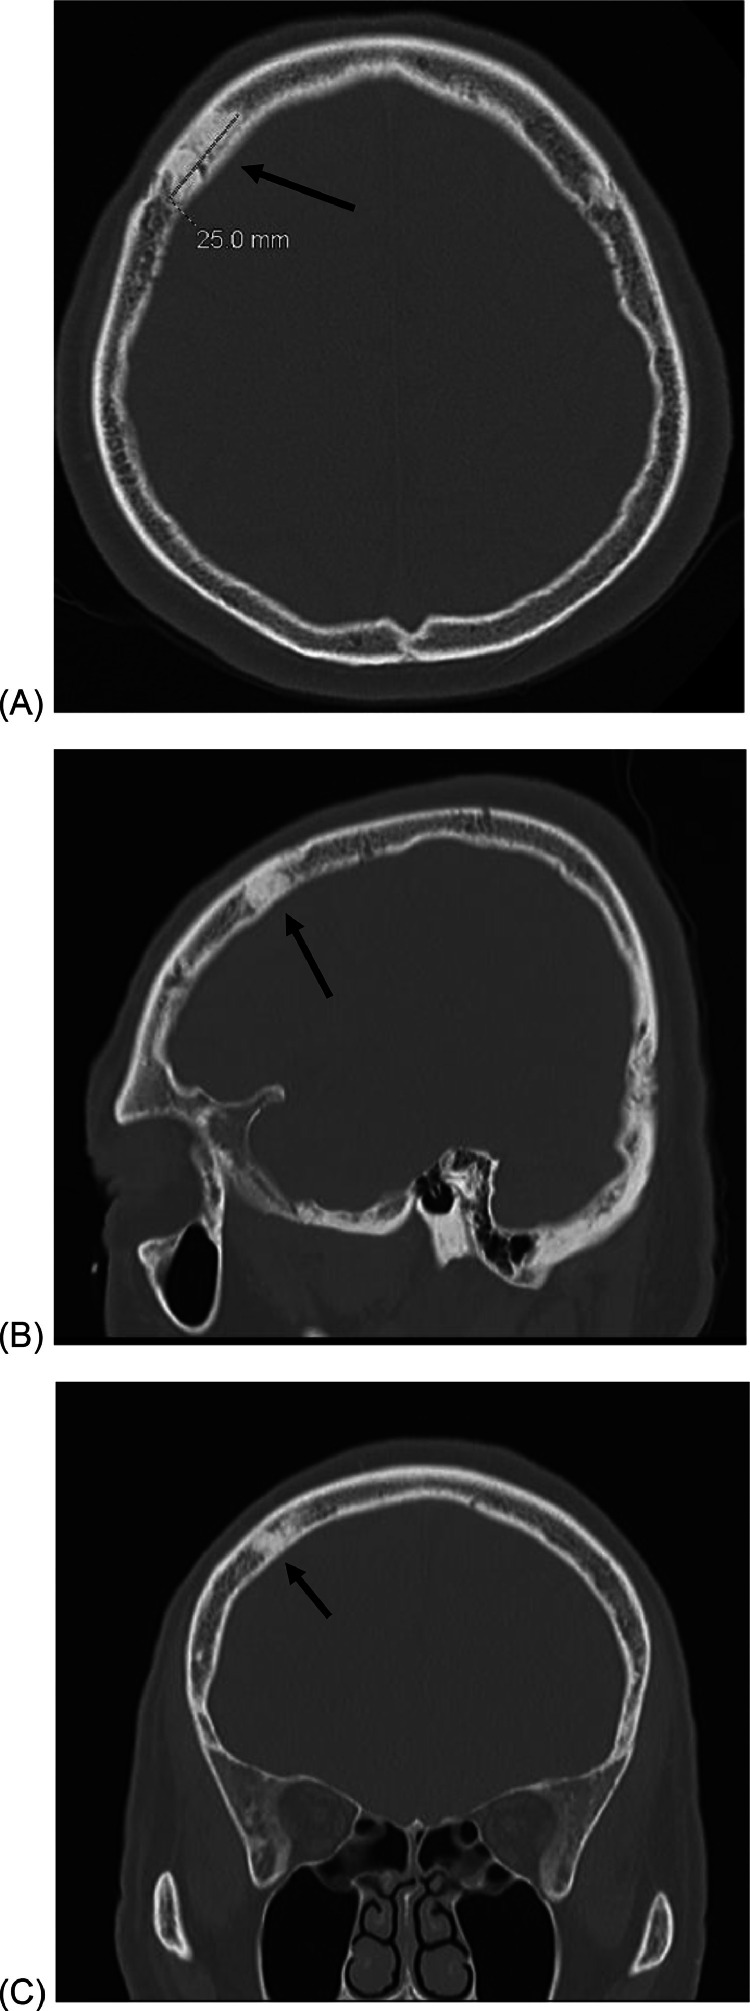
(A) Axial, (B) Sagittal and (C) Coronal CT scan of the suspected skull metastasis.
